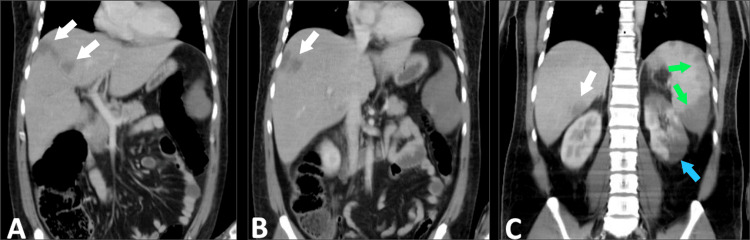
Contrast-enhanced abdominal CT White arrows show infarct areas in segments VIII (A and B) and III (C) of the liver. Green arrows indicate infarct areas in the spleen (C). The blue arrow shows an infarct area in the left kidney (C). CT: computed tomography
